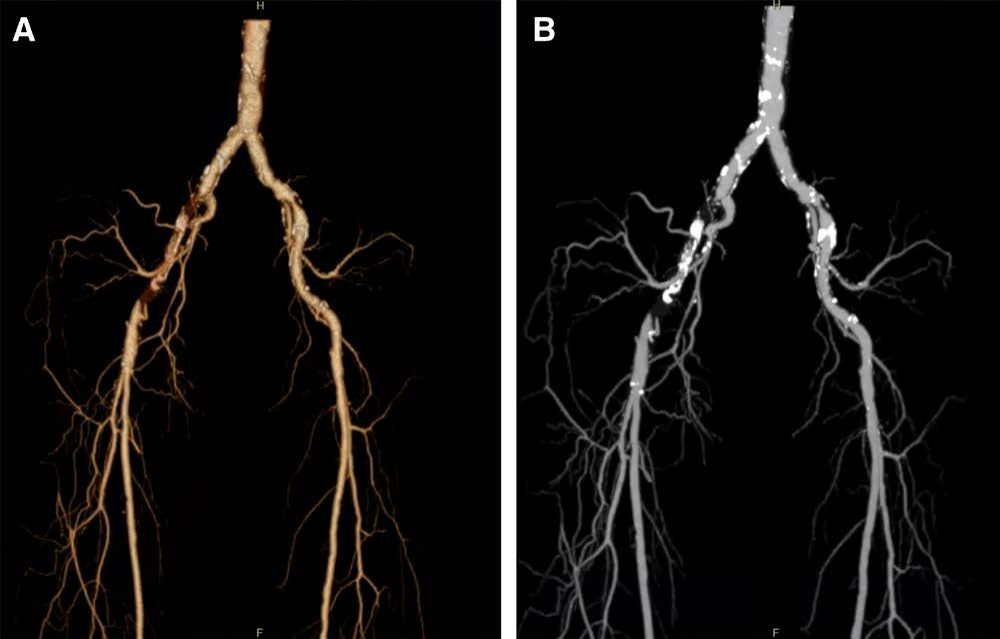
(A) Patient’s local CTA suggests right iliac artery with thrombosis, with no signs of pseudoaneurysm. (B) Maximal intensity projection shows heavily calcified plaque formation. CTA = computed tomography angiography.
